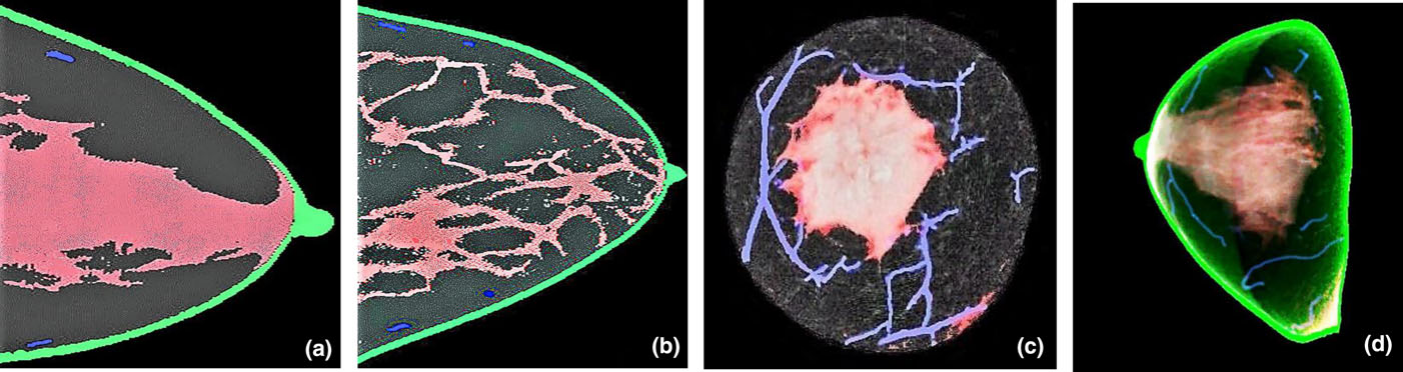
Two examples (a) and (b) of segmented breast CT slices in sagittal view, and two examples (c) and (d) of 3D renderings of an entire BCT image: (c) coronal view with the skin removed for an improved visualization and (d) sagittal view. [Color figure can be viewed at http://wileyonlinelibrary.com]
